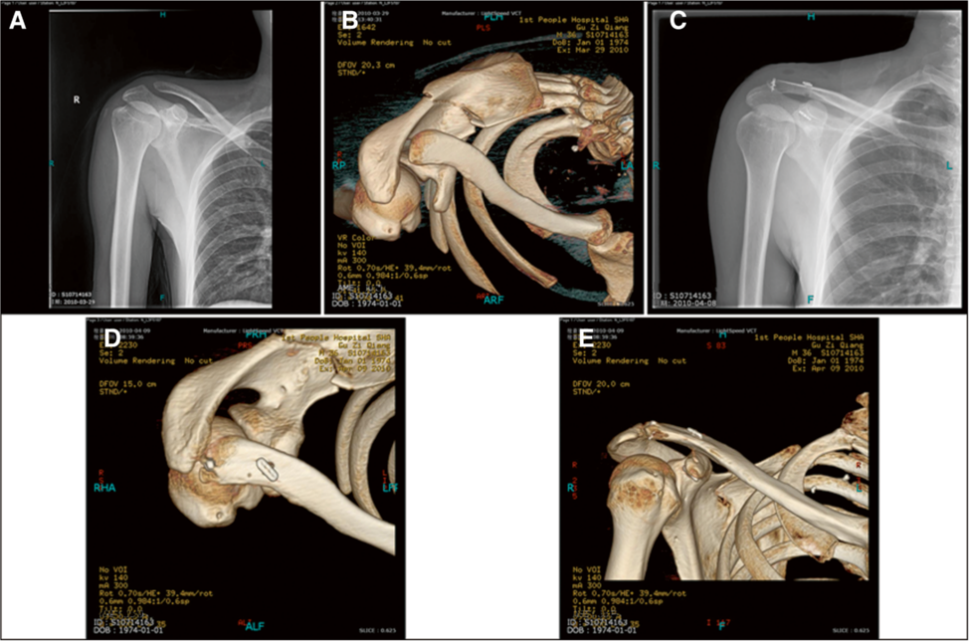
Treatment of Rockwood type IV dislocation. (A) Preoperative anteroposterior X-ray shows acromioclavicular joint space widening. (B) Preoperative CT scan demonstrates that the distal clavicle is displaced backwards, indicating Rockwood type IV dislocation. (C–E) Postoperative X-ray and CT scan indicate that both the vertical and horizontal positions achieve the anatomic reduction.
